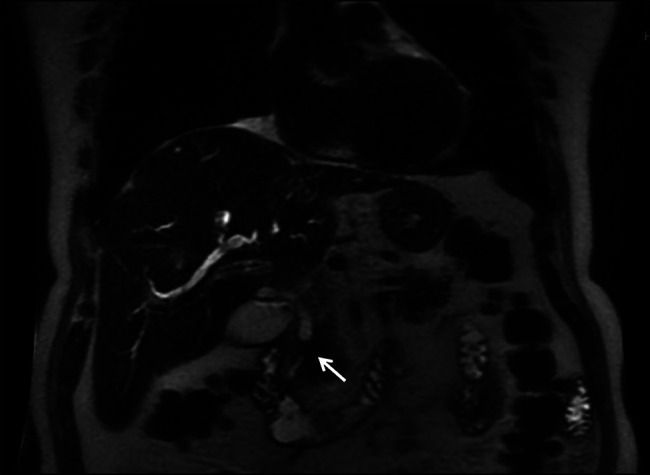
Abdominal magnetic resonance imaging showing moderate intrahepatic and extrahepatic biliary ductal dilatation with the extrahepatic common bile duct measuring 11 mm in the region of the porta hepatis and a 1 cm short-segment stricture (white arrow) involving the distal common bile.
